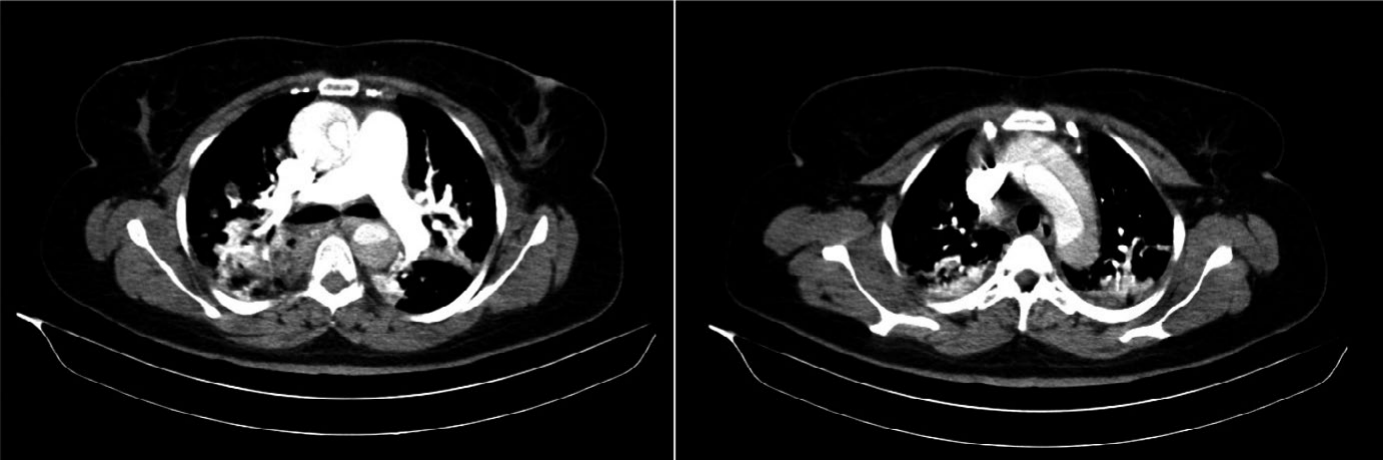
Aortic CT angiography: type A according to Stanford classification and type 1 according to DeBakey classification
